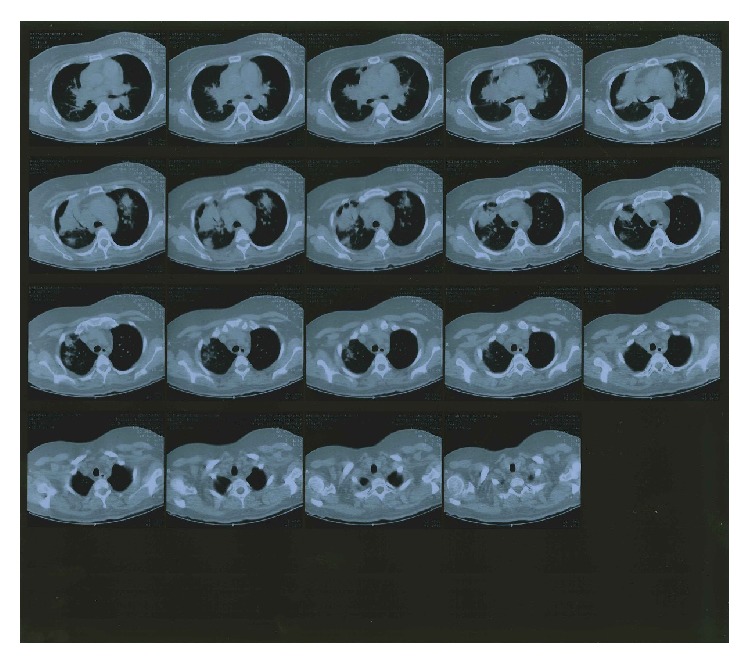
Acute pneumonia caused by* Streptococcus pneumoniae* alone in a 33-year-old female, 6 days after the onset of fever, cough, and dyspnoea. Transverse high-resolution CT image at the level of the right upper lobe shows consolidation, centrilobular nodules, and bronchial wall thickening.
